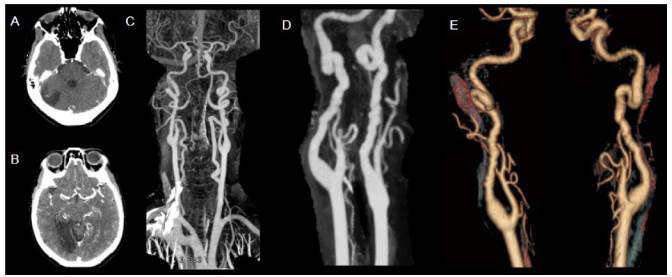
Fibromuscular dysplasia in 60-year-old patient under investigation regarding acute reduction of visual acuity. Axial CT after contrast administration shows infarcted areas in posterior circulation (A and B). CTA with MIP (C and D) and 3D reconstruction (E) show bilateral irregularity and tortuosity of the internal carotid arteries, forming vascular loops in a classic string-of-beads fashion. This classical imaging presentation is not commonly seen in children, who usually have less specific arterial changes that are described as focal or segmental stenosis or occlusions.
